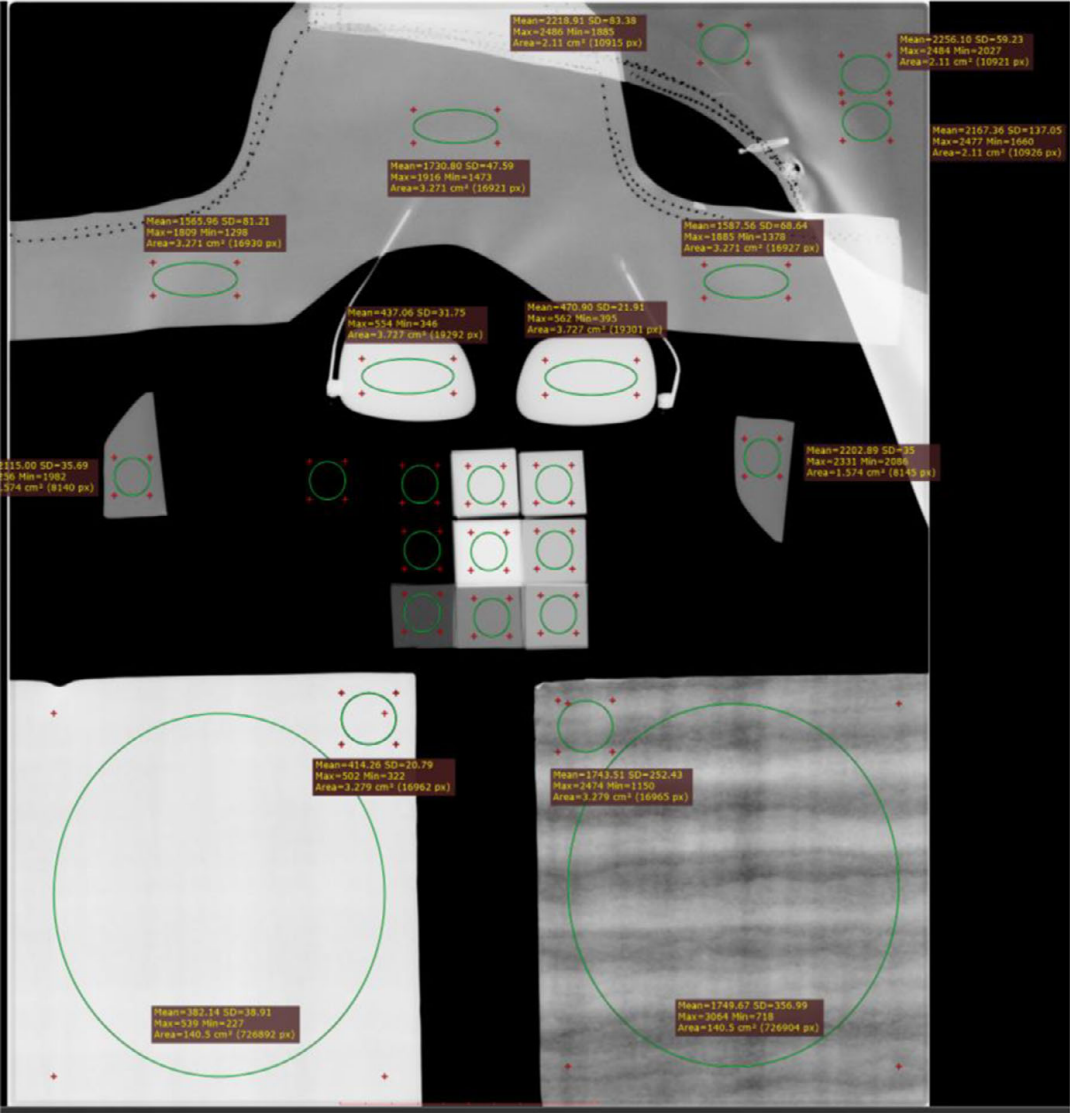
Digital radiographic image of test tool, radiation protection apparel, and lead sheets.
